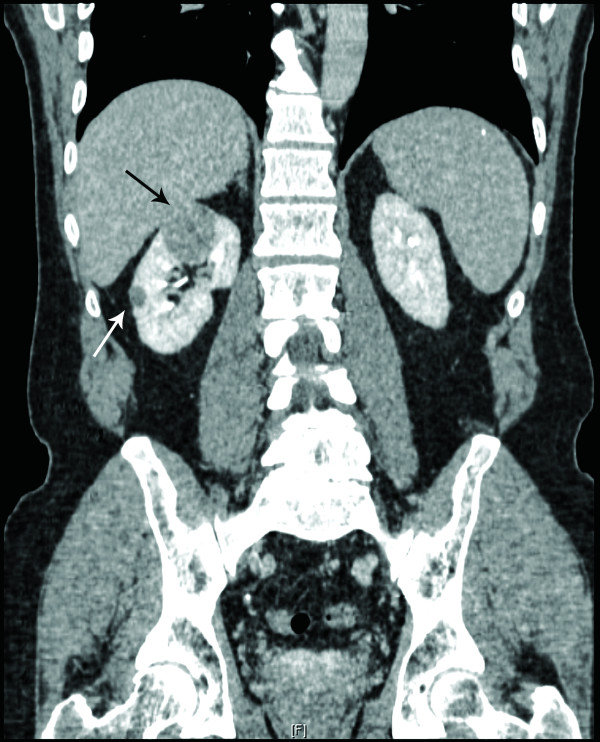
Imaging, Computed tomography (CT) showed the presence of two incidental well-circumscribed masses with similar density in the right kidney. The larger lesion was solid and enhancing and measured 3.5 cm; it was located in the upper pole and approached but did not extend into the renal sinus (black arrow). The smaller lesion measured 1.2 cm and was located along the mid lateral aspect of the kidney (white arrow). The contralateral kidney was normal.
